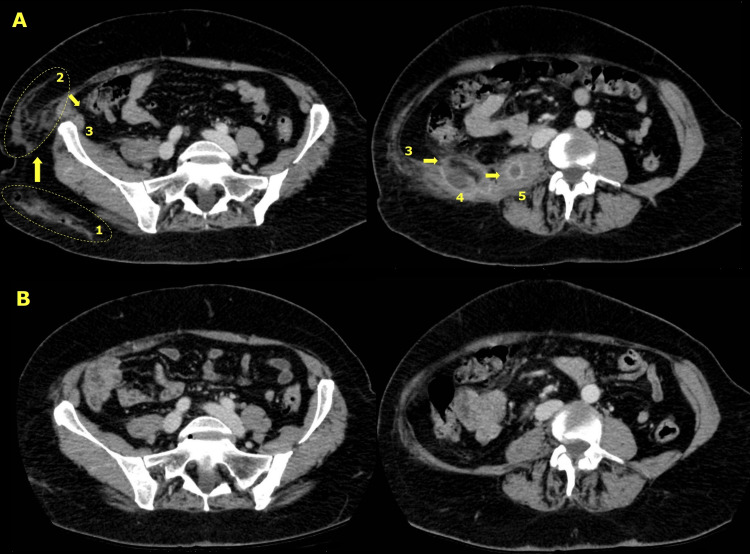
Axial sections of the abdominal CT scans performed on admission (top, A) and three months after discontinuing antibiotic therapy (bottom, B). Upper left, section showing cellulitis in the right buttock (ellipse 1) and adjacent (ellipse 2) to the right posterior abdominal wall (3). Upper right, section showing abscesses in the right quadratus lumborum (4) and psoas (5) muscles. The numbers and arrows indicate the likely route of dissemination. At the bottom, the same sections showing the full resolution of the lesions seen at the top.
